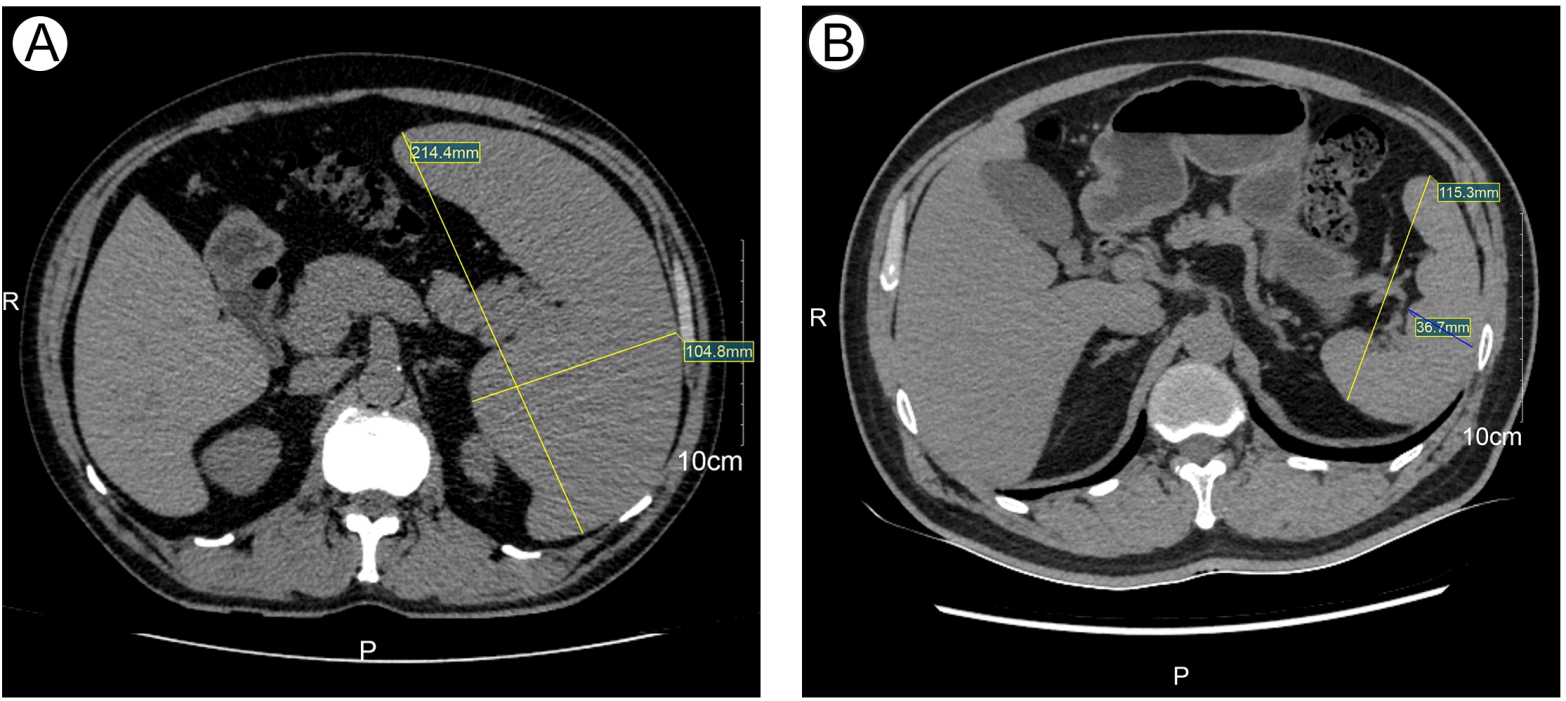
The size of Spleen at initial diagnosis and after treatment detected by CT. (A) Spleen size at initial diagnosis detected by CT. (B) Spleen size after treatment detected by CT.
